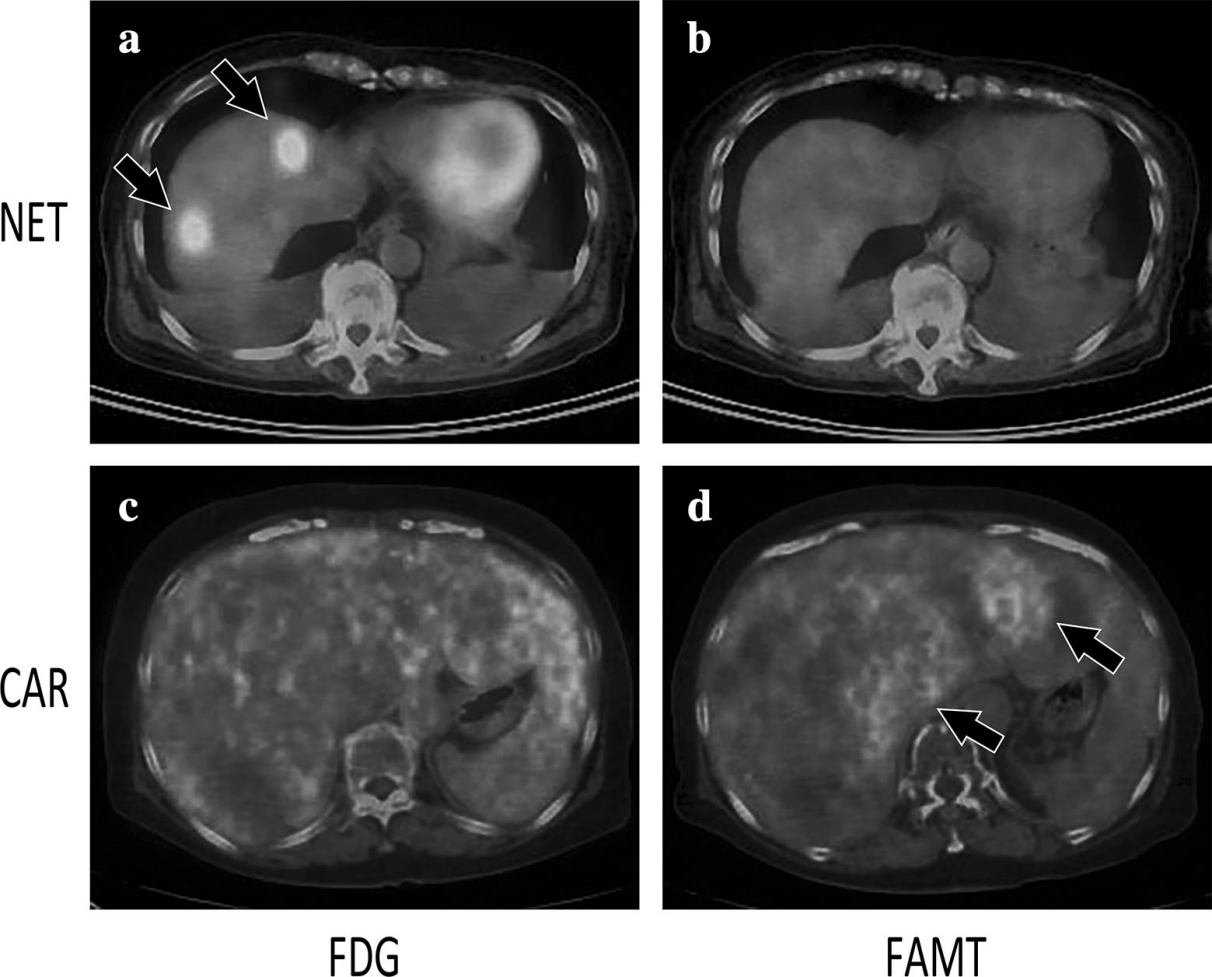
PET/CT fusion images with either FDG or FAMT administration for the same patients with NET or CAR. For a patient with NET, FDG PET images showed high spotty accumulations (*arrow*) in the liver (a). No FAMT uptakes were seen in the liver (b). For a patient with CAR, FDG PET showed diffuse uptake for normal liver parenchyma without uptakes for tumor lesion in the liver (c). FAMT PET showed some faint massive uptakes (*arrow*) of FAMT in the liver (d). *FDG* 2-[^18^F]-fluoro-2-deoxyglucose, *FAMT* L-3-[^18^F] fluoro-alpha-methyl tyrosine, *T/L* tumor-to-liver parenchymal, *CAR* carcinoid tumor, *NET* neuroendocrine tumor and pheochromocytoma
